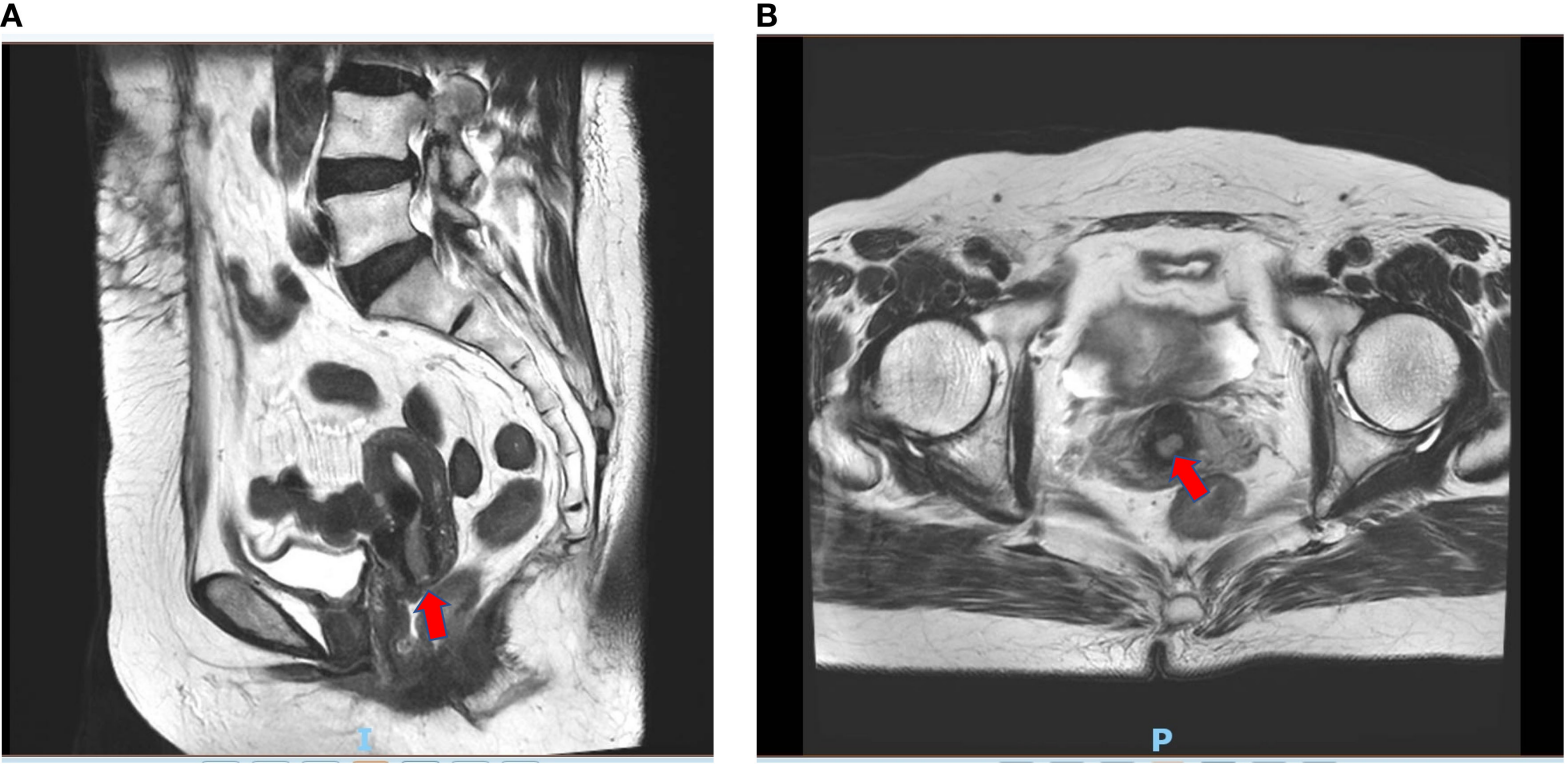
Enhanced magnetic resonance imaging of suspected cervical lesion on April 20th 2024. (A) Sagittal view of enhanced magnetic resonance imaging of suspected cervical lesion on April 20^th^ 2024. (B) Axial view of enhanced magnetic resonance imaging of suspected cervical lesion on April 20^th^ 2024.
